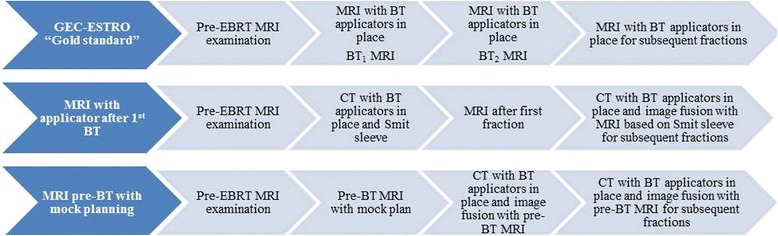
Work flow with incorporation of MRI with the GEC-ESTRO “gold standard” and 2 alternative approaches for limited MRI availability with MRI after 1^st^ BT and MRI pre-BT with mock planning
